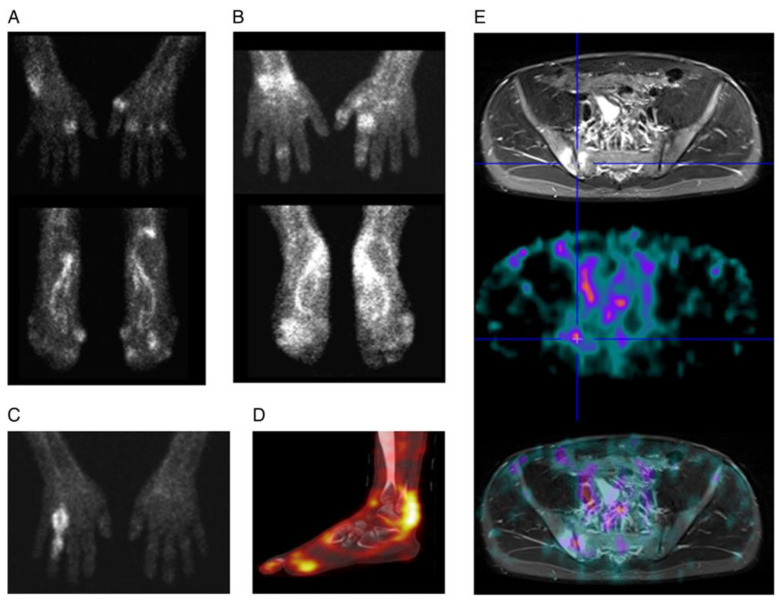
Certolizumab pegol labeled with Tc99m was distributed in the hands, feet, and sacroiliac joints 4-5 h after injection. (A) A patient with active rheumatoid arthritis exhibited a polyarticular pattern of joints without involvement of distal interphalangeal joint (DIP). (B) Left second digit distal interphalangeal joint uptake in polyarticular psoriatic arthritis patients. (C) Tracer uptake in dactylitis patient of the fourth digit. (D) SPECT-CT scan of a patient’s right foot showing Achilles tendon enthesitis and spondyloarthritis. (E) Fusion of sacroiliac joint SPECT and MRI images in an axial spondyloarthritis patient. Reprinted from open access source (CC BY-NC-ND 4.0) [194].
